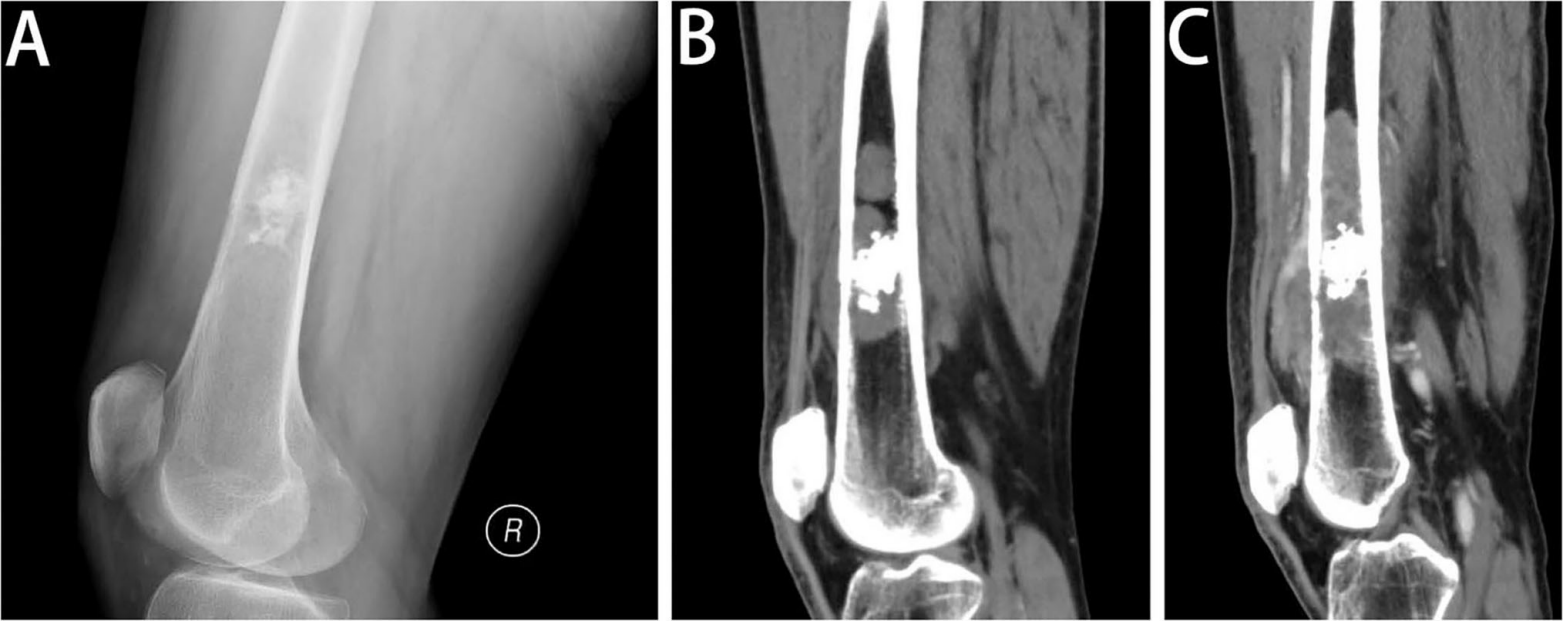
Radiologic features of DCCS. (A) Lateral view of the left femur. Radiography of the distal femur shows a lesion in the medullary cavity with calcifications, with subtle destruction of the anterior cortex and anterior soft tissue mass. (B) Sagittal CT scan of the left femur in the soft tissue window. The sagittal CT images demonstrate that the lesion is multifocal, with penetration of the cortex and considerable soft tissue components. In addition to the calcific foci, the lesion contains more areas of lytic destructive changes. (C) Sagittal post-contrast CT scan of the left femur in the soft tissue window. One and a half months later, the sagittal post-contrast CT image in the soft tissue window shows the lesion expanding into a large area of bone destruction, with heterogeneous enhancement.
